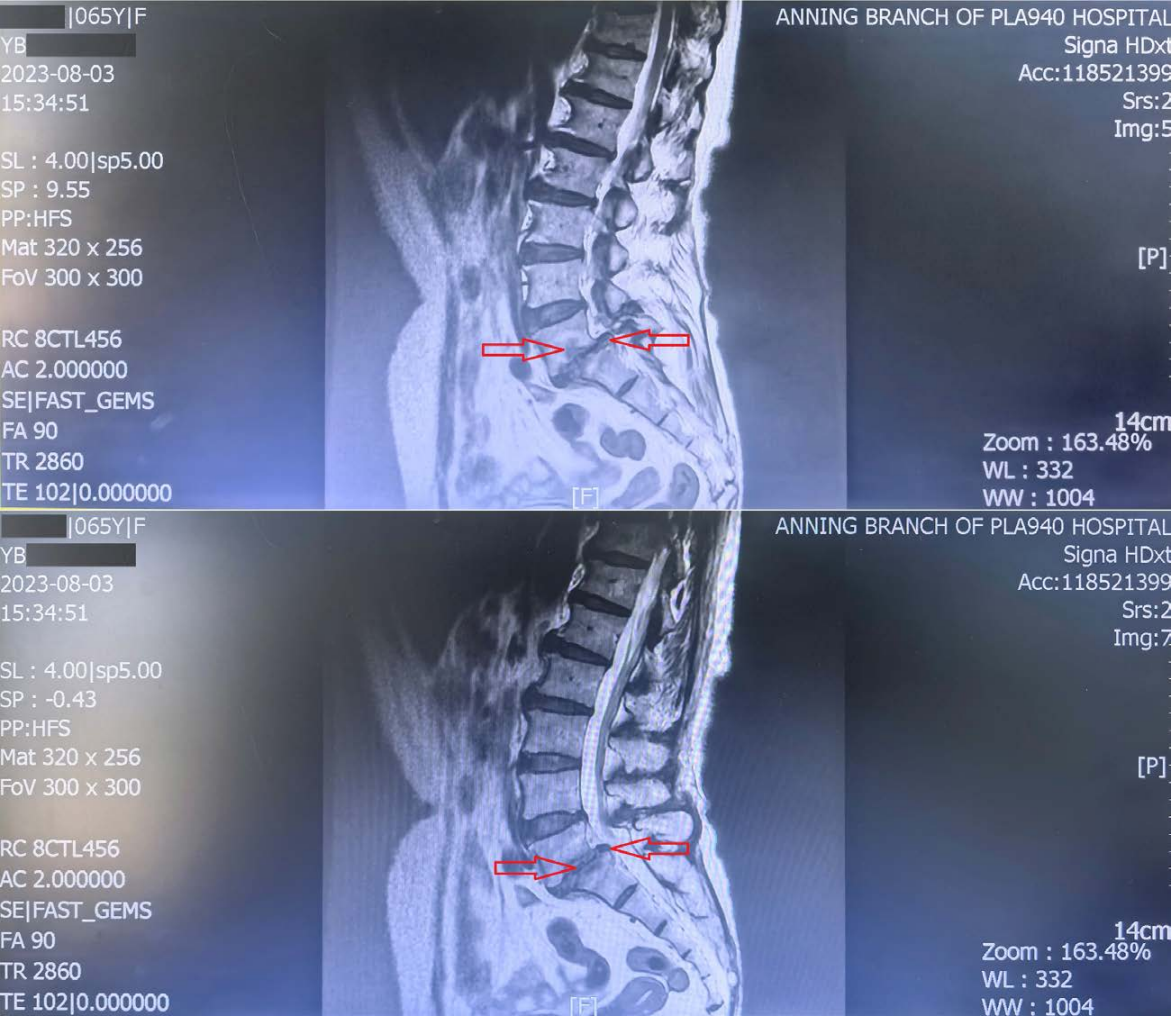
Magnetic resonance image of the patient’s lumbar spine. The red arrow indicates the site of the lesion: the lumbar magnetic resonance imaging showed abnormal signals in the body of L5 and S1 vertebrae and the L5-S1 intervertebral space, and slight effusion in the subcutaneous soft tissue of the lumbar and sacral region. L5 = fifth lumbar vertebra, S1 = the first sacral vertebra.
